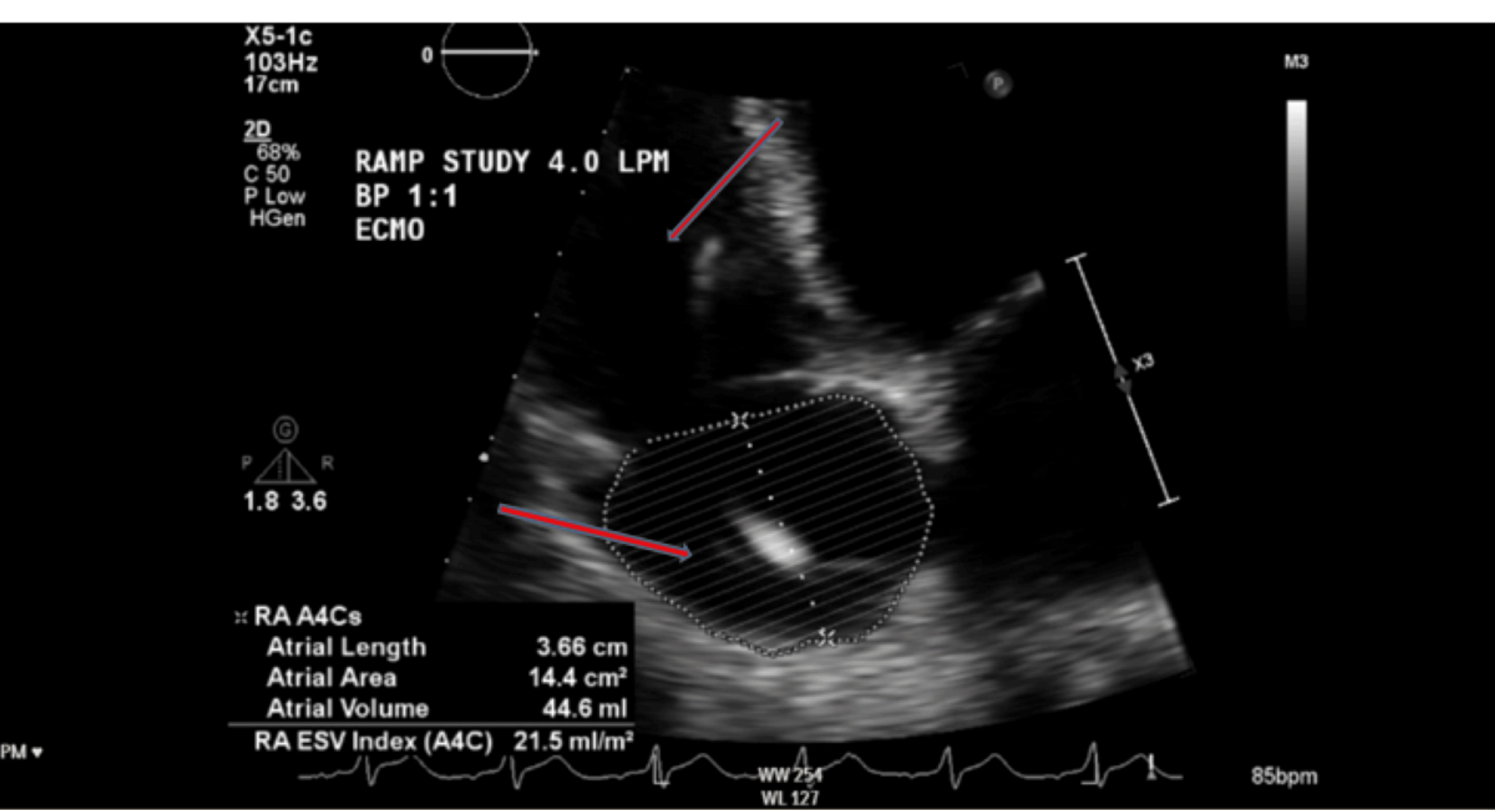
TTE apical four-chamber view showing a markedly dilated right atrium and ventricle TTE: transthoracic echocardiogram
